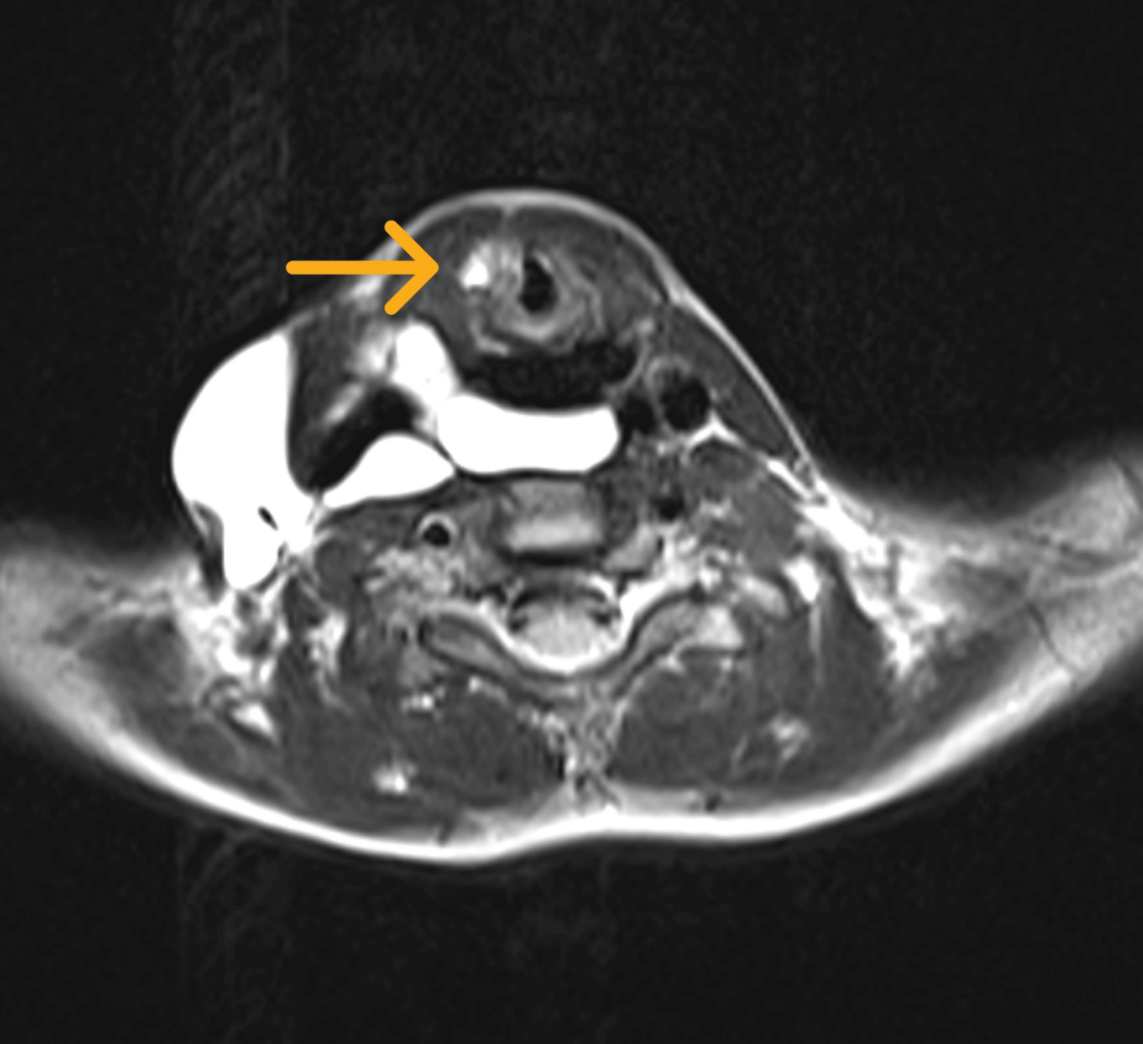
An axial view of the MRI neck shows an enhancing cystic lesion in the retropharyngeal region insinuating into the right carotid space and encircling the right sternocleidomastoid. The right aryepiglottic fold shows a hyperintense signal (arrow) and is displaced medially.
